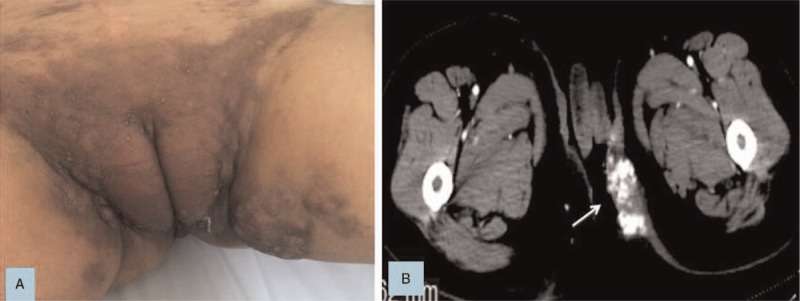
A. Vulva nodules was larger than before. B. CT scan revealed that vulvar tumor was significantly larger after multiple lines of conventional therapies and calcification was visible inside.
